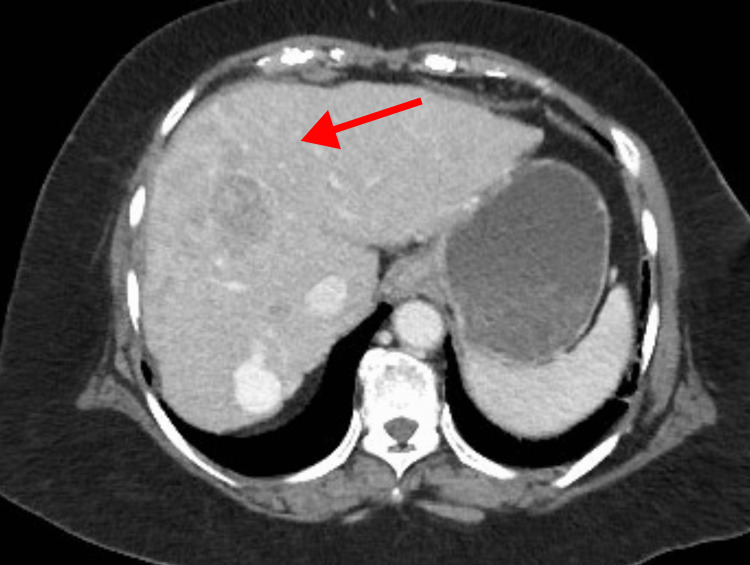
Venous phase of triphasic CT scan The image shows a contrast-enhanced CT scan of the liver in the venous phase, showing lesions exhibiting rapid relative washout. The red arrow highlights a hypodense lesion consistent with the imaging characteristics of hepatocellular carcinoma.
